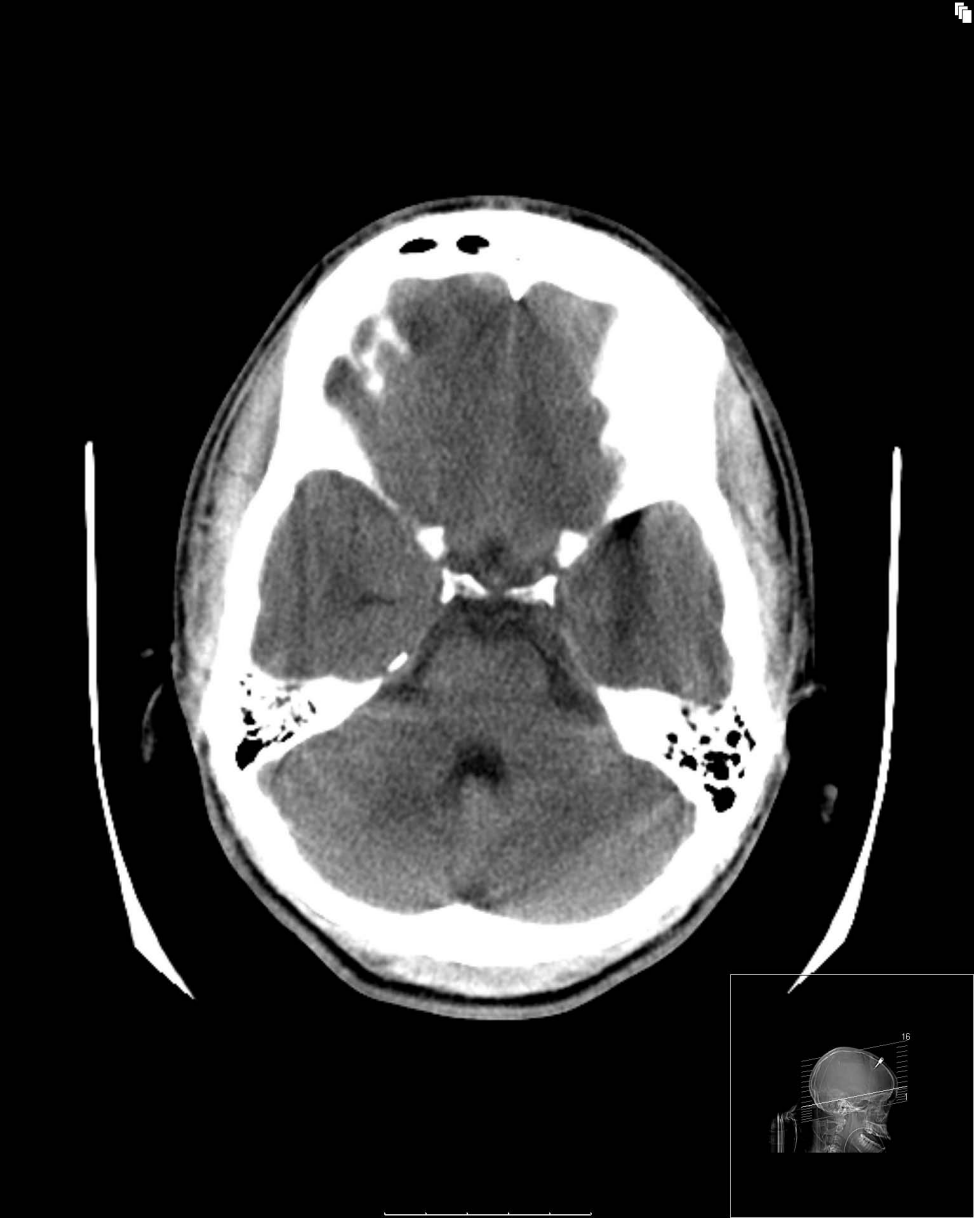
Computed tomography image showing relative sparing of the infratentorial compartment.
